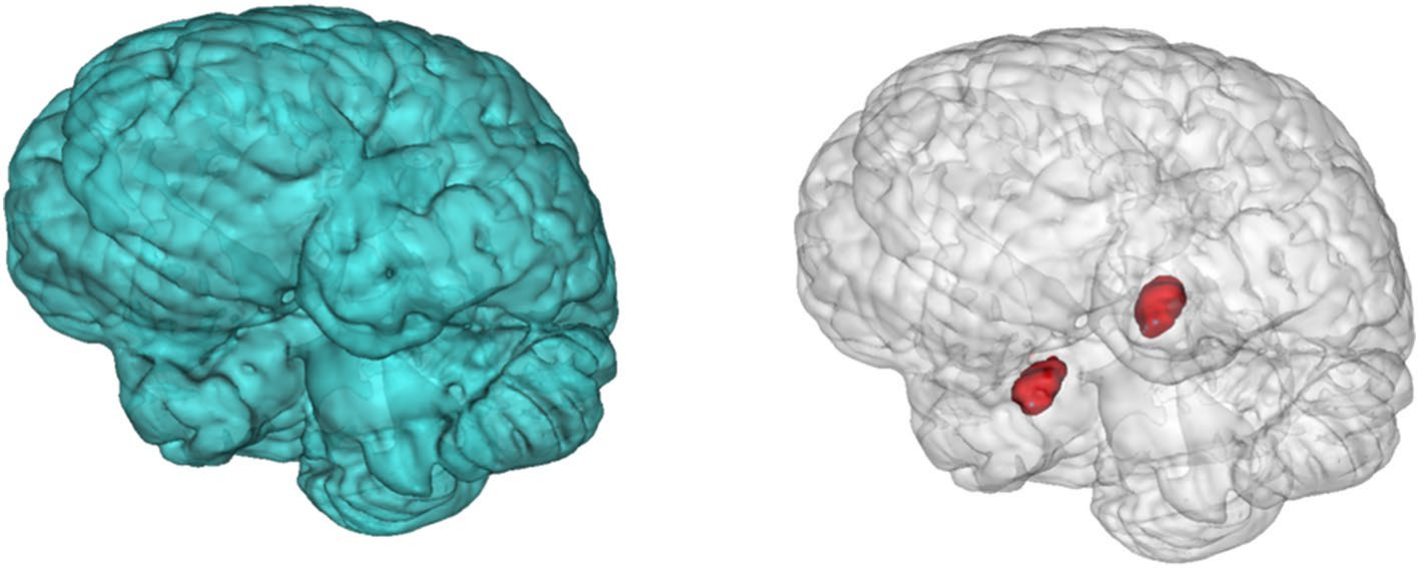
T1-weighted MRI scan showing the total brain (in blue) and amygdala (in red).
